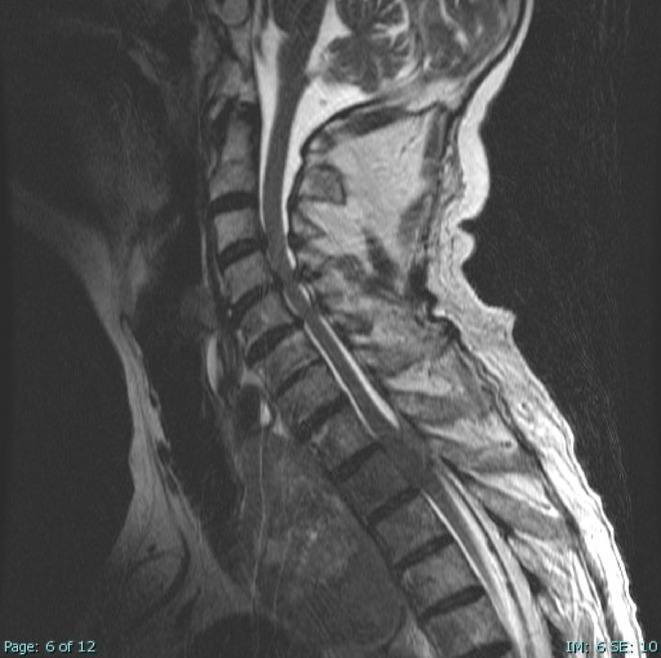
FIGURE 2 Spine MRI showing a large mass with signs of bone invasion of the vertebral bodies from D1 to D4, extension into the intraspinal region at these levels and compression of the spinal cord.
